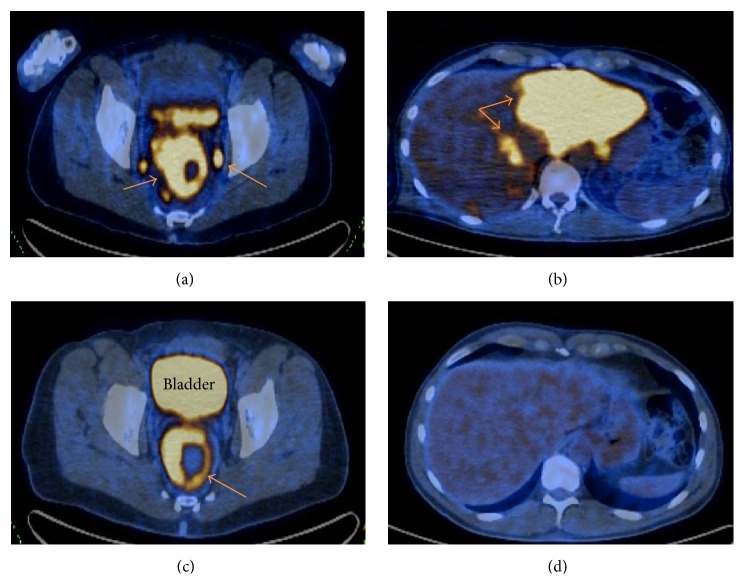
PET/CT scan showing intense FDG avid. (a) Rectal mass and surrounding lymphadenopathy. (b) Multiple liver lesions at diagnosis. (c) Interval partial response of the rectal mass to therapy. (d) Complete metabolic resolution of liver lesion and posttreatment changes noted after 6 cycles of chemotherapy.
